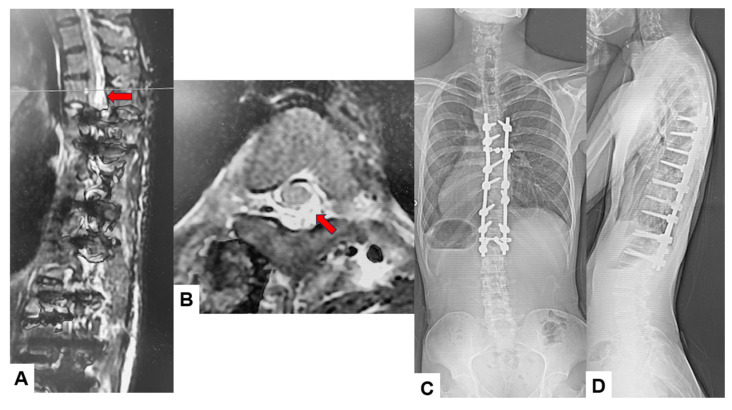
True TcMEP-positive case. 18-year-old male, AIS, Postoperative MRI and revision surgery. Red arrows indicate an acute spinal epidural hematoma. (A) Postoperative T2-weighted sagittal MR imaging, (B) postoperative T2-weighted axial MR imaging at T4, (C) final posteroanterior radiogram, and (D) final lateral radiogram.
